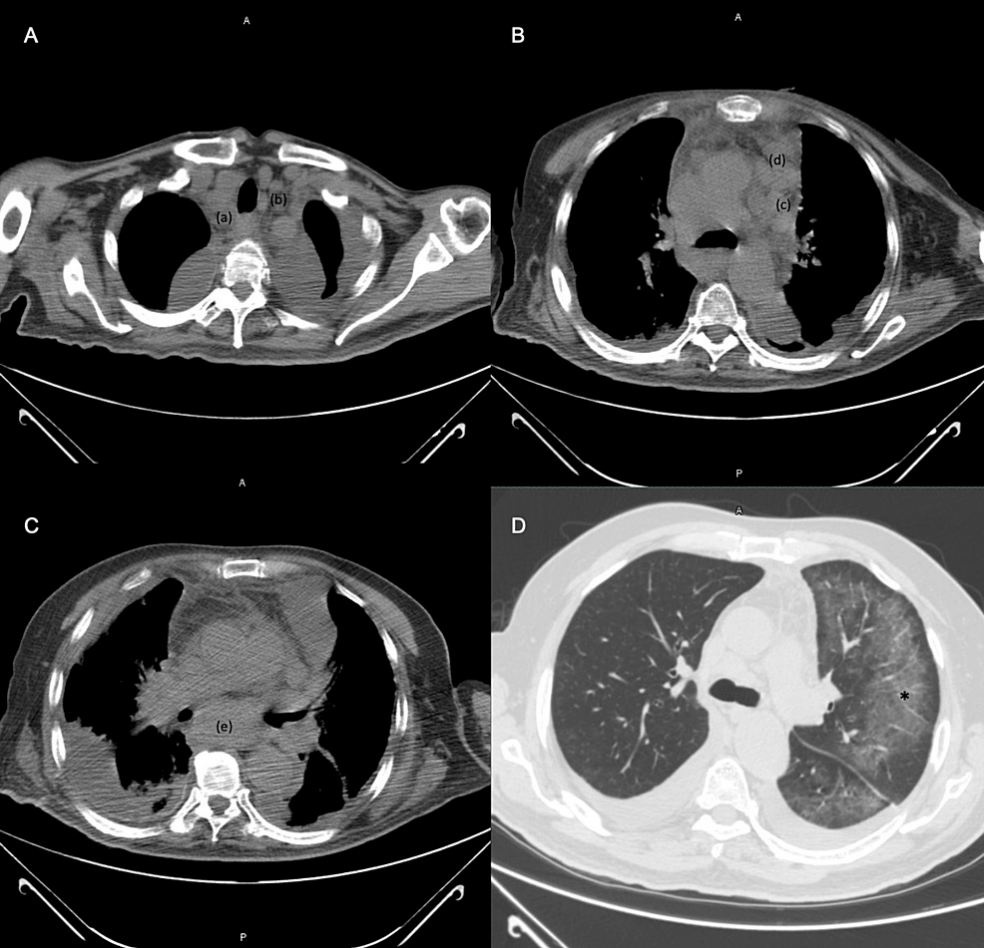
Simple chest CT conducted after thoracentesis with the mediastinal window setting A: The presence of lymphadenopathy is seen in regions 2R (a) and 2L (b), as well as bilateral pleural effusion; B: Lymph nodes in regions 5 (c) and 6 (d); C: Adenopathy in region 7 (e) and bilateral pleural effusion; D: Ground glass (*) seen in the left axial interstitium of the subpleural region
